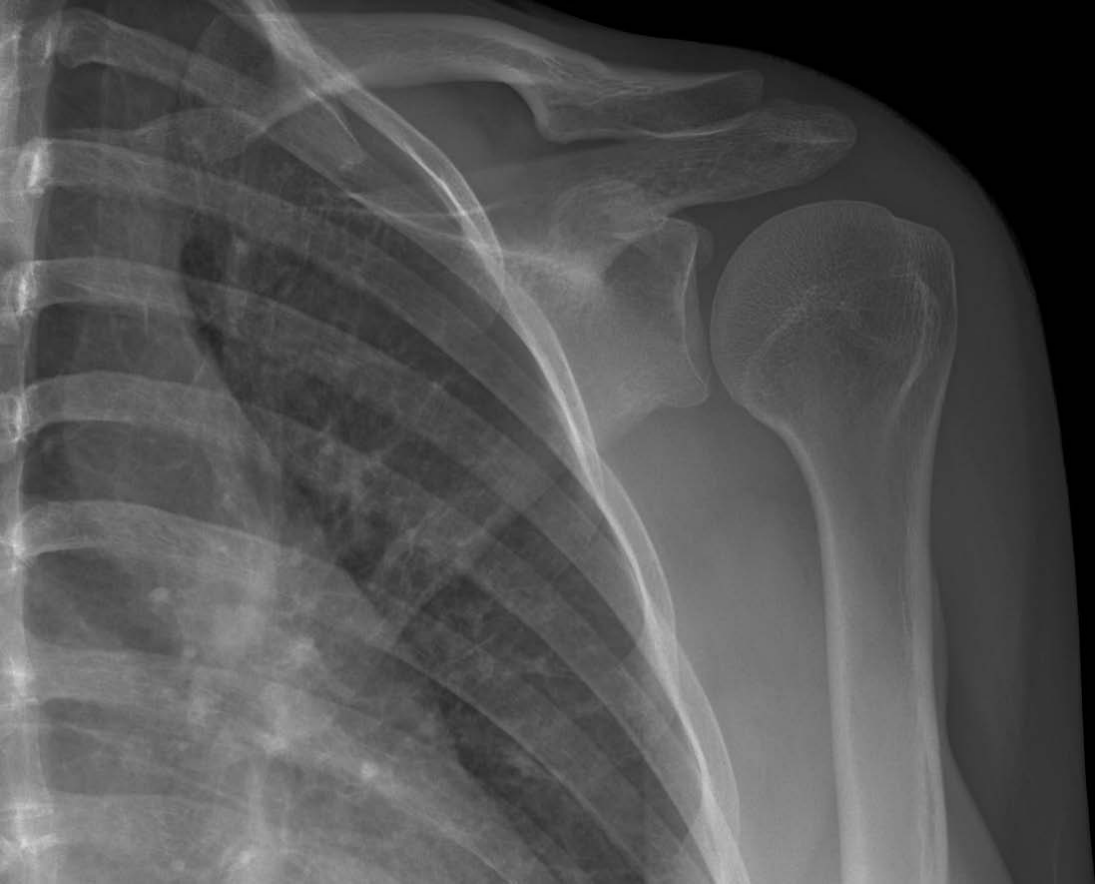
AP radiograph of the nondisplaced pathologic coracoid process fracture.
